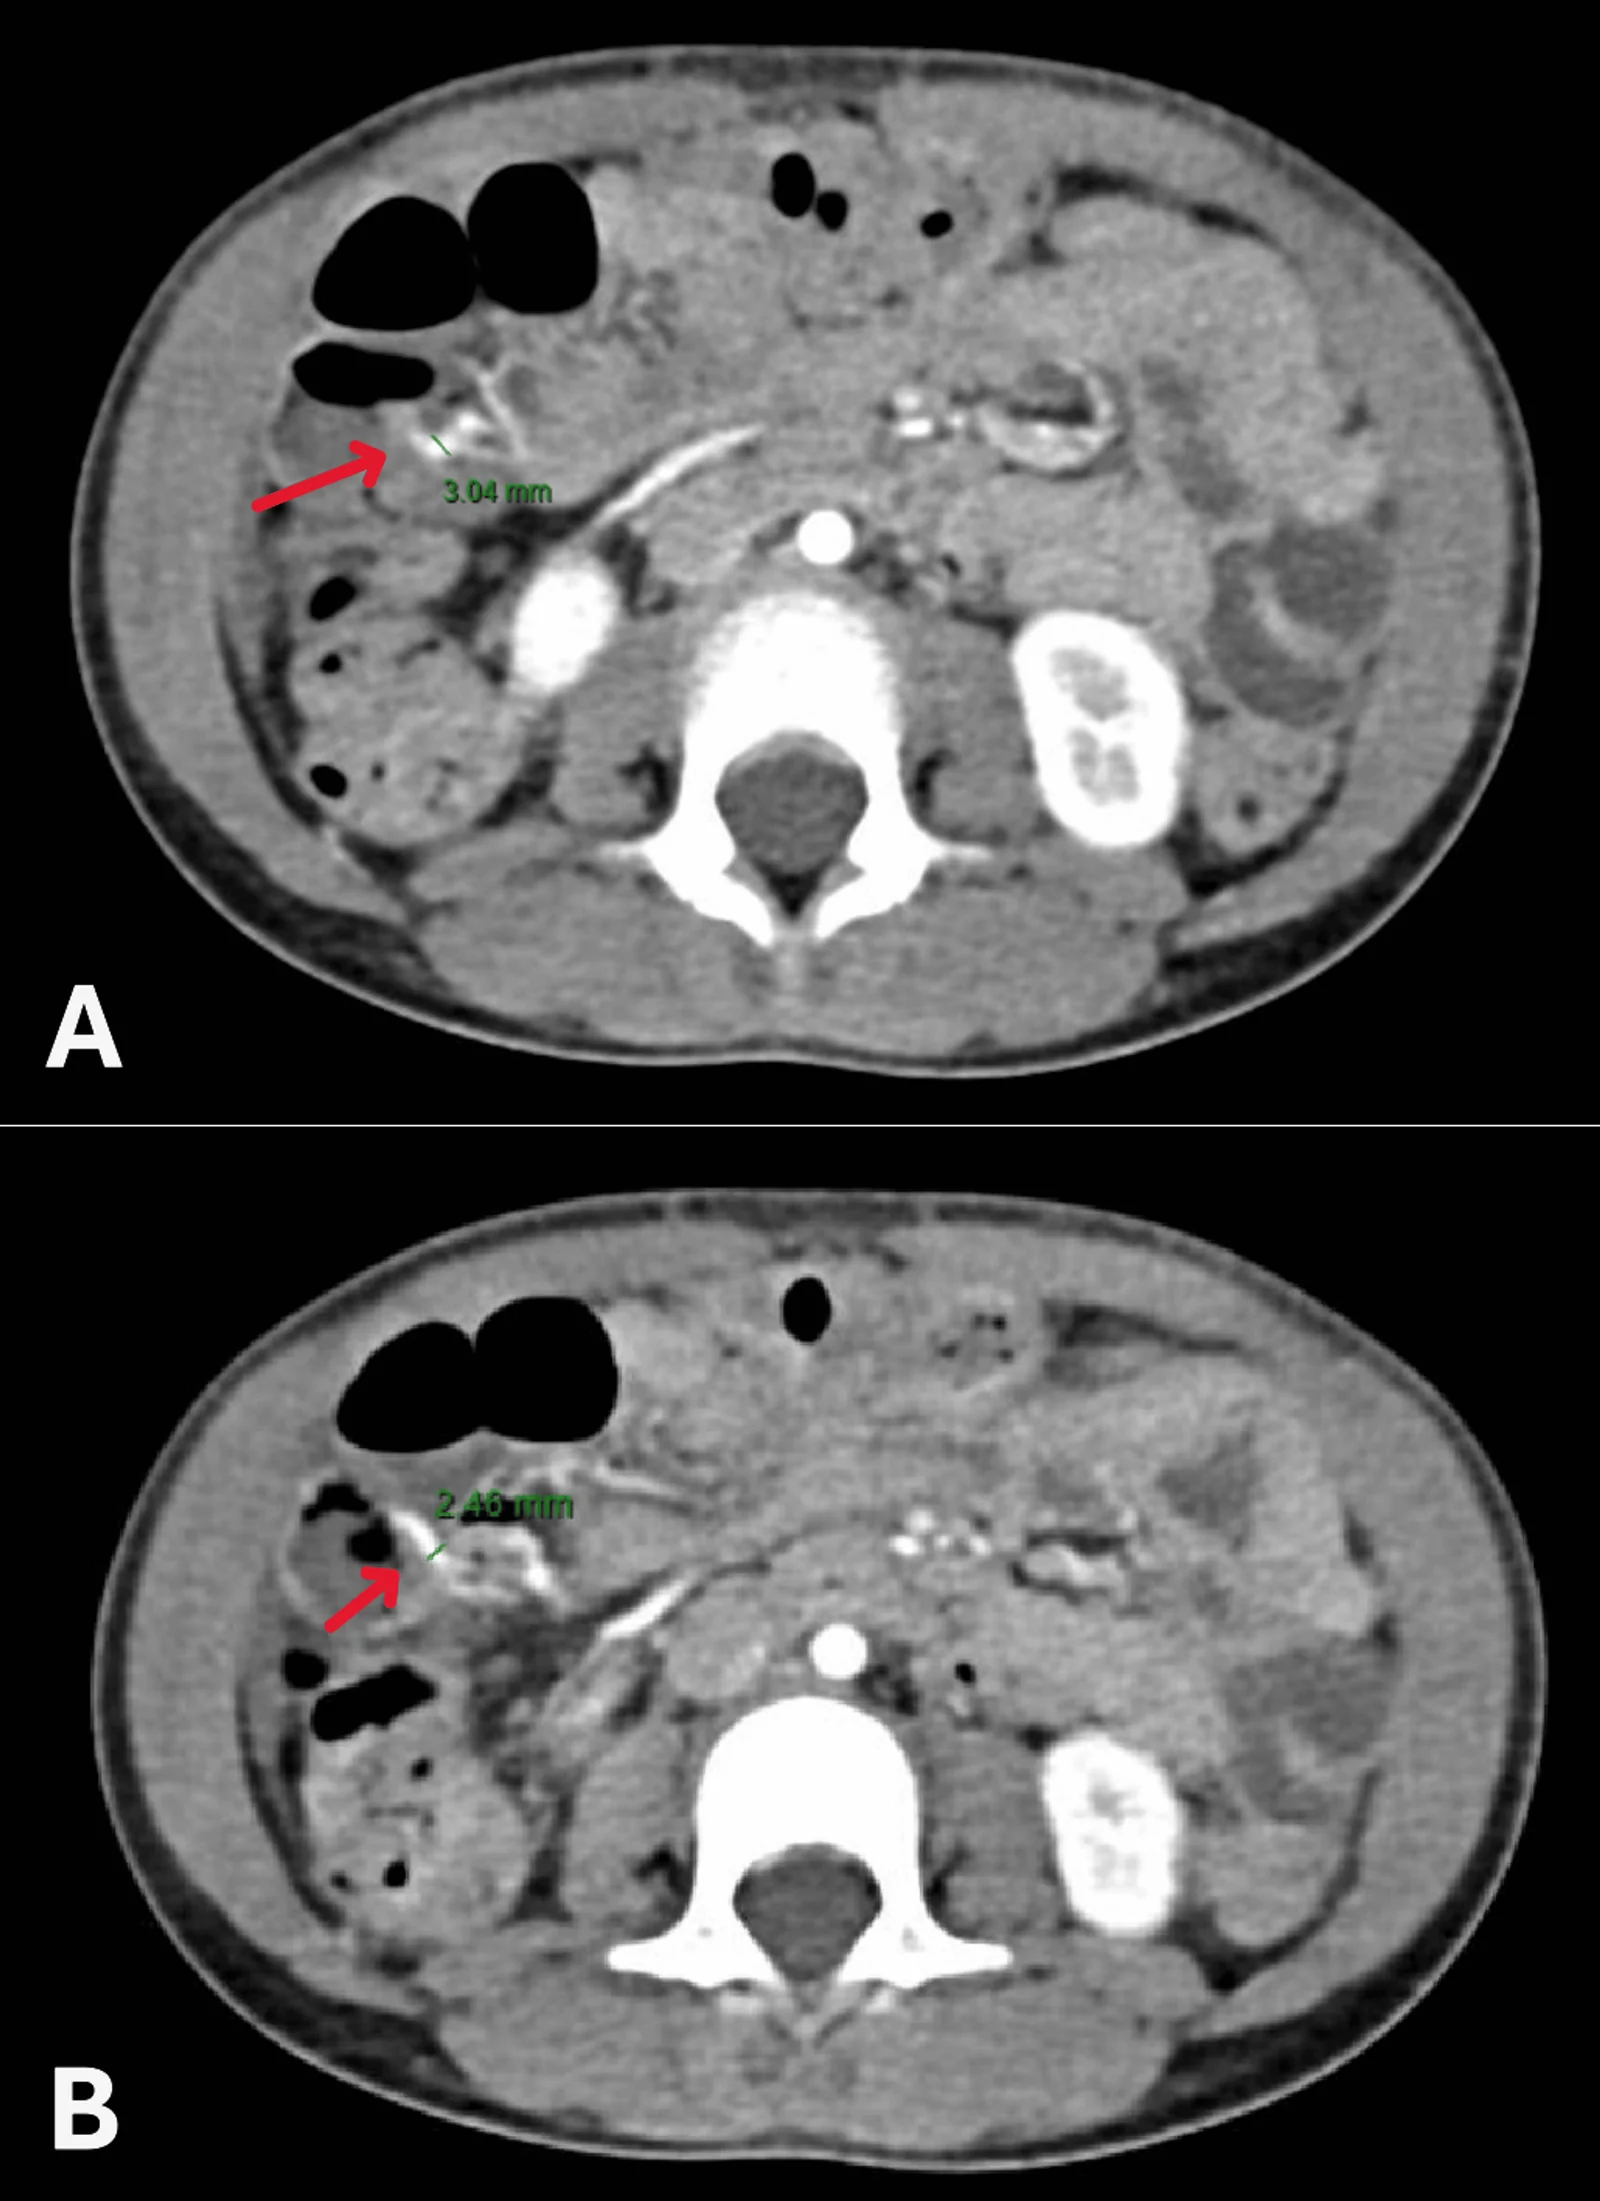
Contrast-enhanced CT angiogram of the abdomen and pelvis demonstrating prominent mesenteric vessels in the right abdomen. (A) Axial CT angiogram demonstrating prominent enhancing vessels in the right abdomen, consistent with the hepatic flexure varix identified on colonoscopy (red arrow). (B) Adjacent axial CT angiogram demonstrating continuity of the varix (red arrow).
